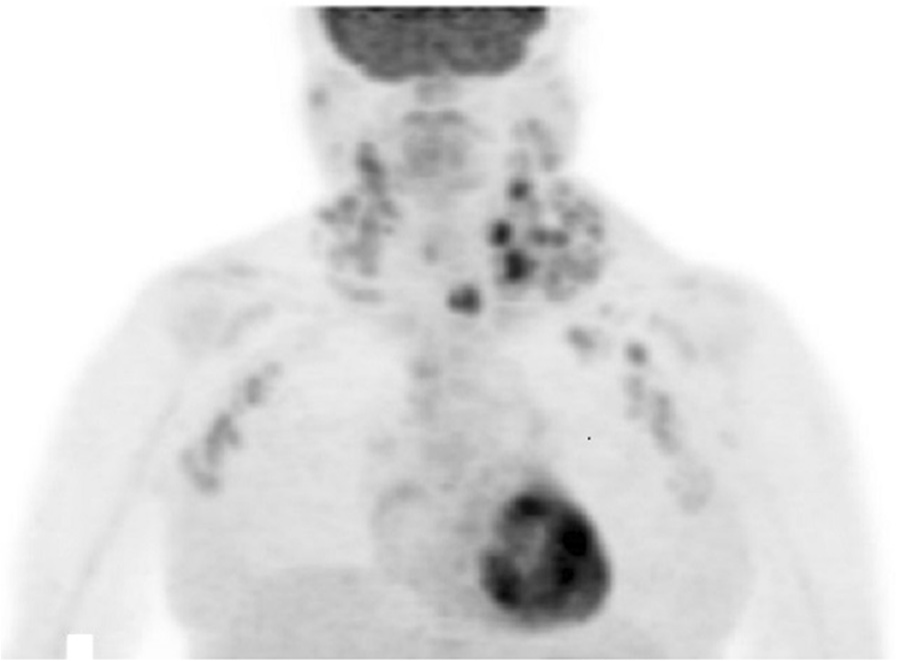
FDG-PET/CT imaging demonstrated higher FDG uptake in cervical and supraclavicular lymph nodes
